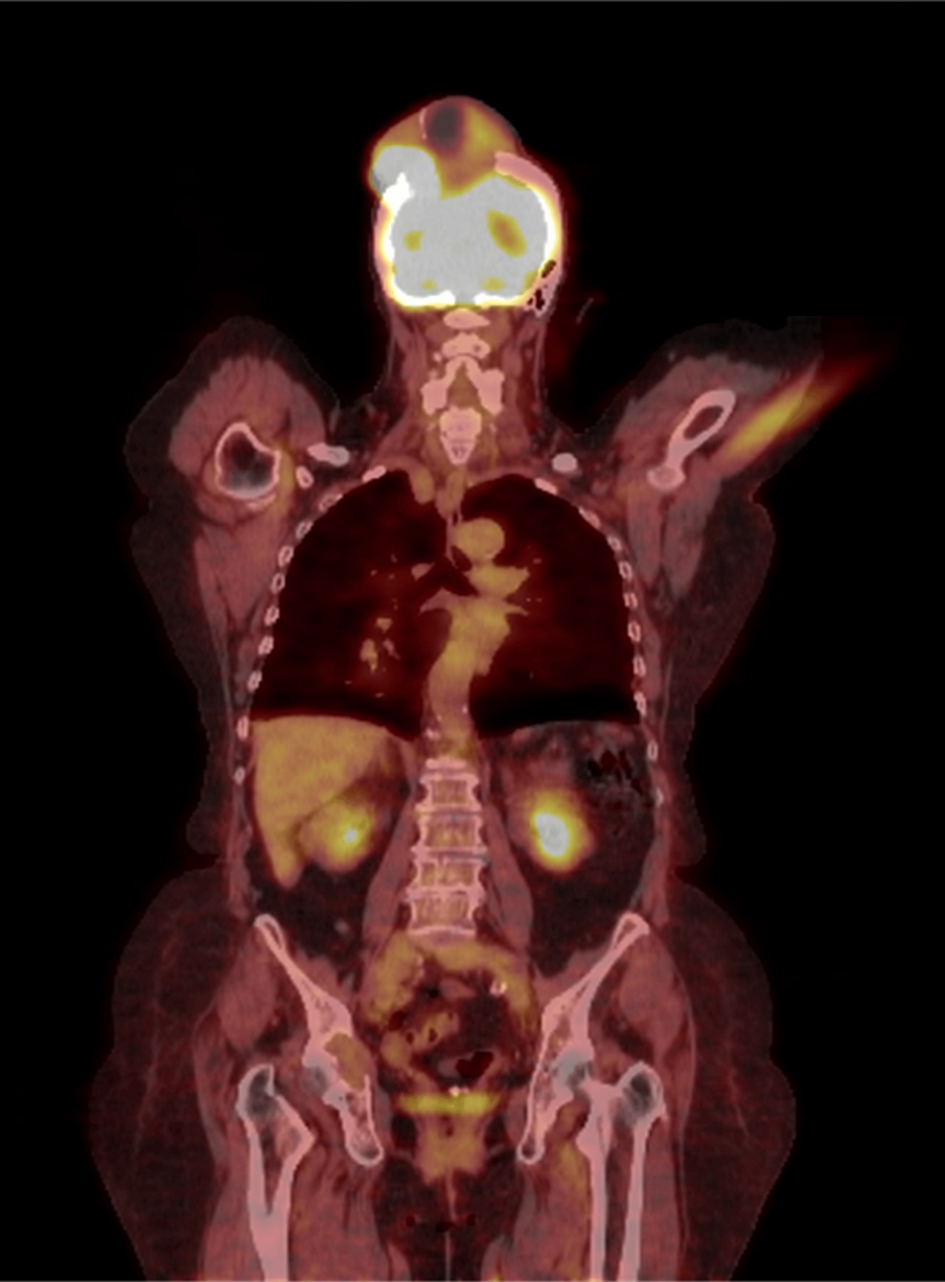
Whole-body PET/CT scan. The PET/CT scan revealing a calvarial mass with significant bony destruction and additional calvarial lesions. PET/CT, positron emission tomography/computed tomography
